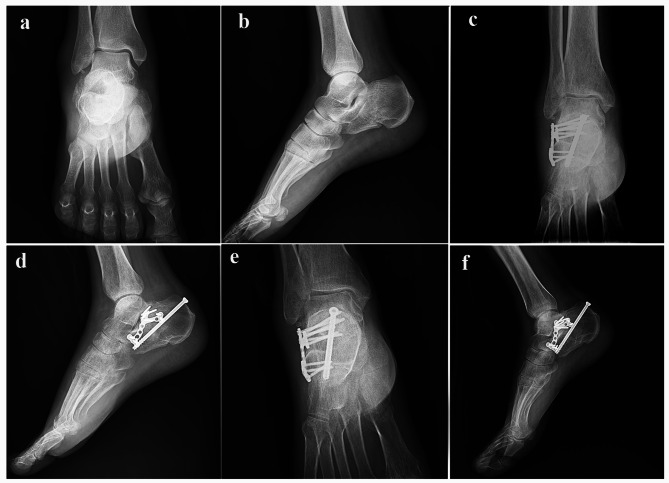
(a and b) Preoperative anteroposterior and lateral radiographs of the ankle; (c and d) anteroposterior and lateral radiographs of the ankle immediately after plate fixation; (e and f) anteroposterior and lateral radiographs of the ankle immediately after plate fixation
